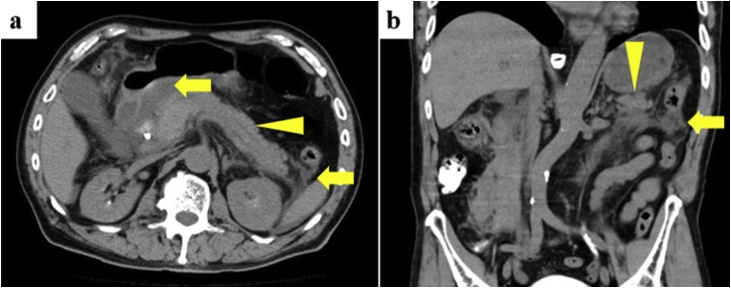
Contrast enhanced abdominal computed tomography scan revealed increased density of fat tissue (arrow) around the pancreas (arrowhead). a axial view, b coronal view.
